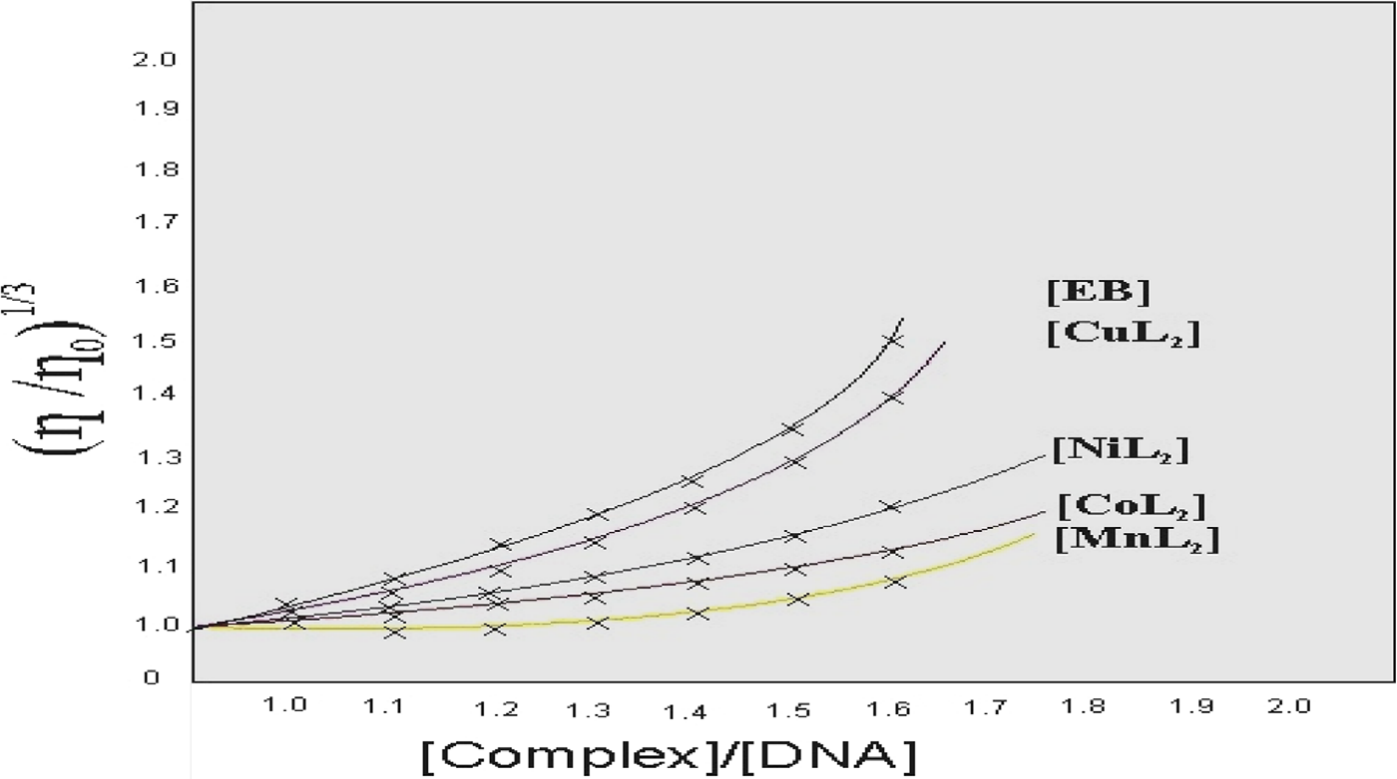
Effect on relative viscosity of CT-DNA under the influence of increasing amount of.
